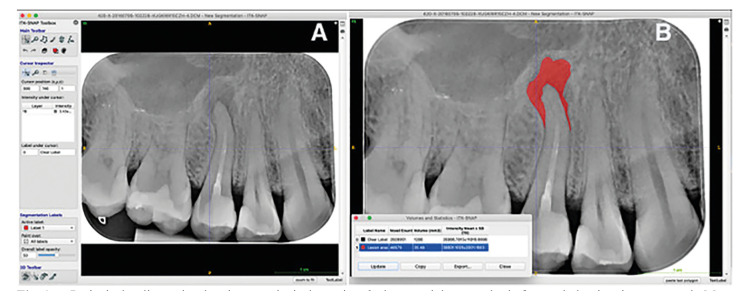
a. Periapical radiography showing a periapical area in a 2nd upper right premolar before endodontic microsurgery. b. Measurement performed manually in the program Itk-Snap (20.49 mm2).
